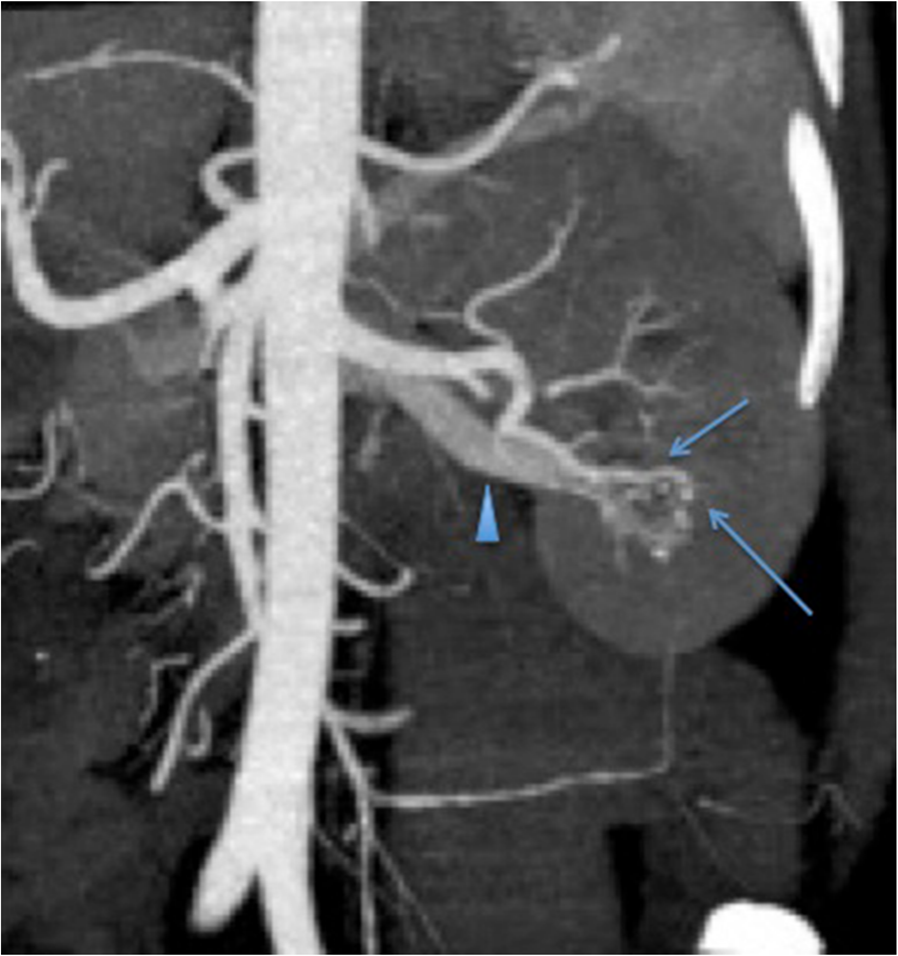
Sagittal oblique multiplanar reformat image of the computed tomography angiogram shows early filling of the left renal vein (arrowhead) and several hypertrophic peripheral renal artery branches in the lower pole of the left kidney (arrows), this is consistent with a renal arteriovenous malformation.
